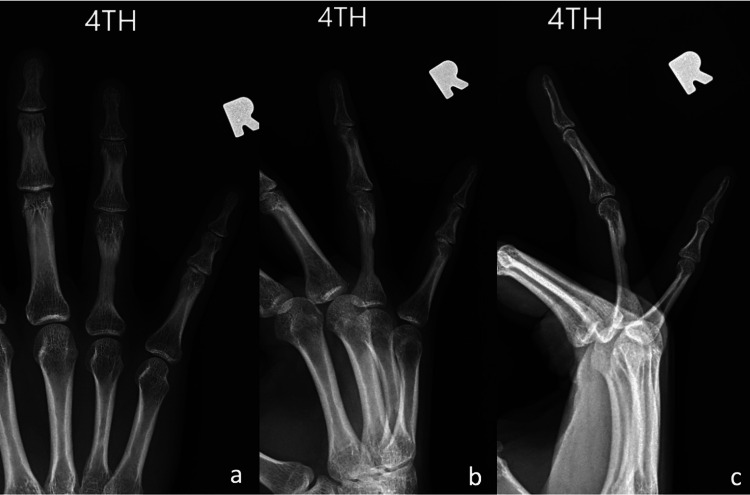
Radiographs six months postoperatively demonstrating improved cortical density of the proximal phalanx.
